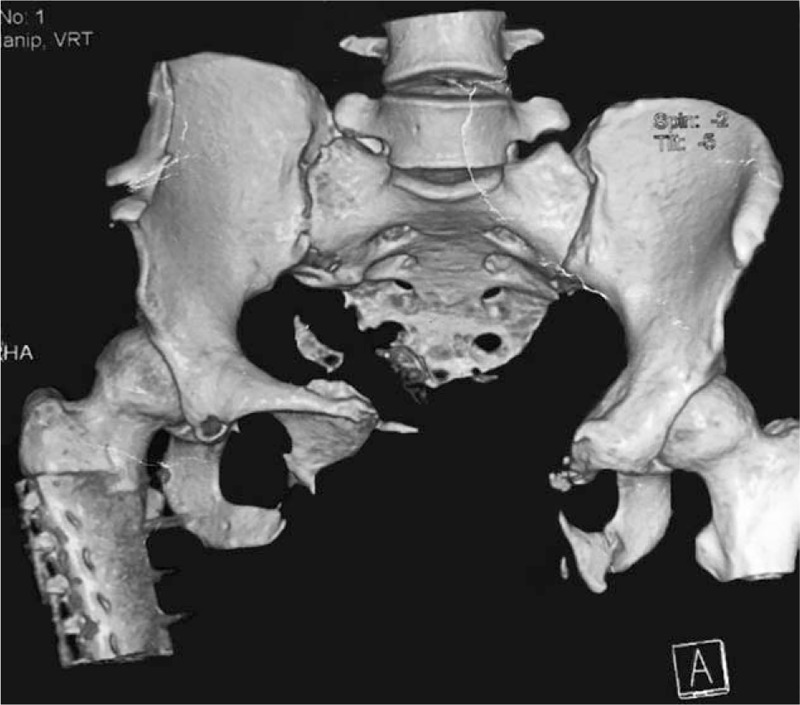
Computed tomography combined with 3-dimensional reconstruction shows the comminuted pelvic fracture, severe diastasis of the pubic symphysis, and the internal fixation change of the right femoral shaft fracture.
